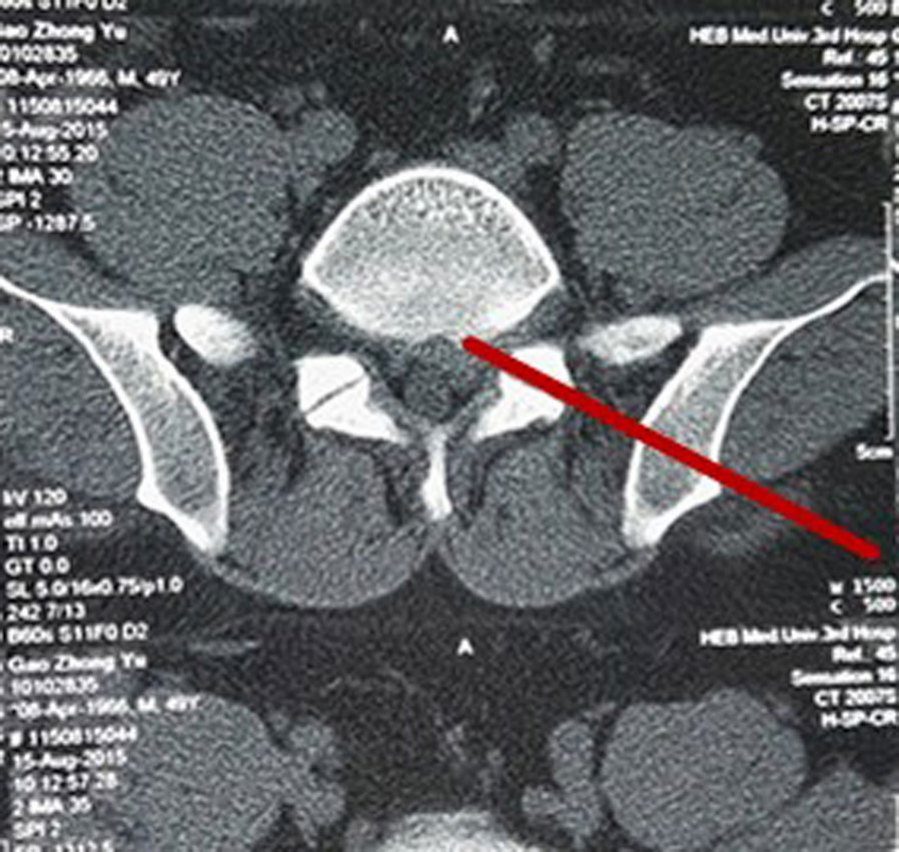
According to preoperative CT of the surgery segments to plan a good channel approach to foramen (the *red line*)
